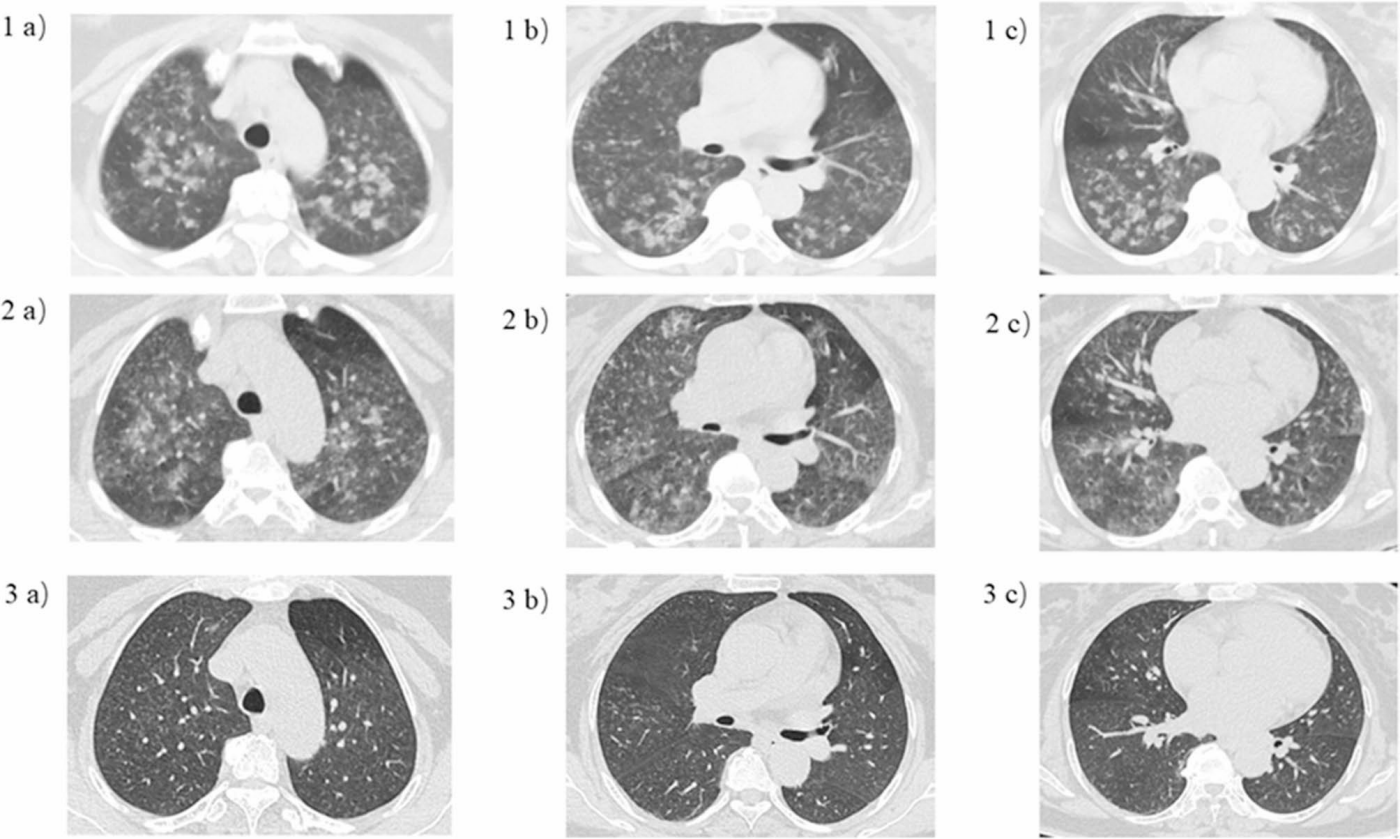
Comparison of chest CT with the same plain layer at different times. (1a-1c) Chest CT 8 h after inhaling nitric acid fumes: Diffuse exudation can be seen in upper, middle and lower lungs. (2a-2c) After inhaling for 3 days: Diffuse exudation is similar to before. (3a-3c) After inhaling for 5 days: the exudation is obviously absorbed than before
